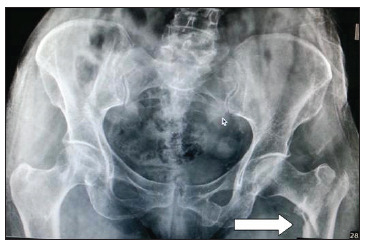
Preoperative radiograph showing Evan's type 4 left intertrochanteric hip fracture (white arrow).
